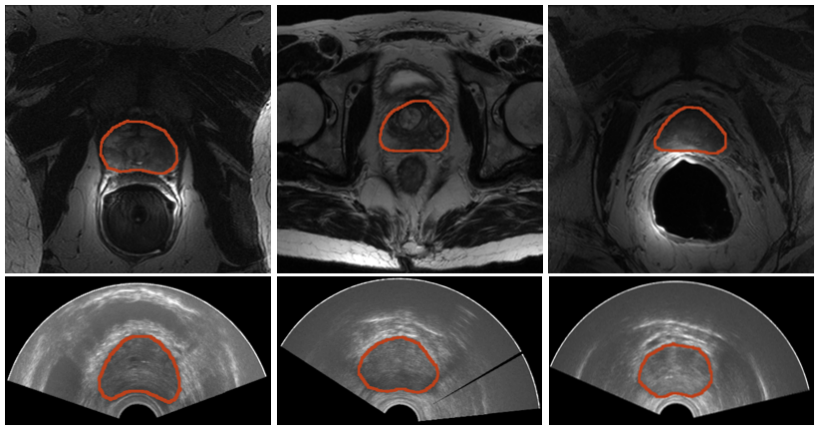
Samples of images from both modalities. (Top) Prostate MRI. From left to right, a sample image for each of the datasets—PROMISE12, SAML, and ZENODO—is shown. (Bottom) Three sample prostate TRUS from the ZENODO dataset.
